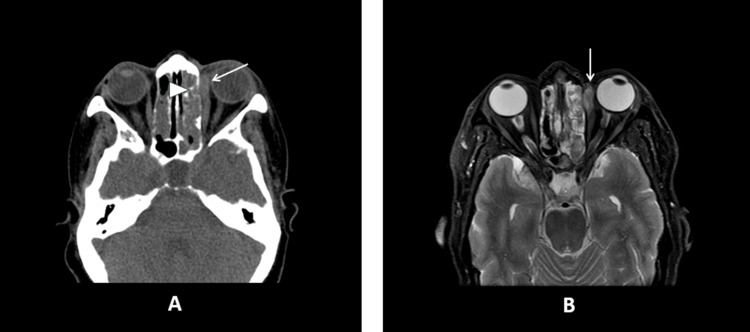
First orbital CT and MRI with contrast. (A) Axial CT image showing heterogeneous high to intermediate attenuation of the left medial rectus muscle (straight arrows). There is questionable dehiscence of lamina papyracea (arrowhead). (B) Axial T2-weighted MR image showing heterogeneous high signal intensity of the anterior left medial rectus indicating intramuscular hematoma (straight arrows).
